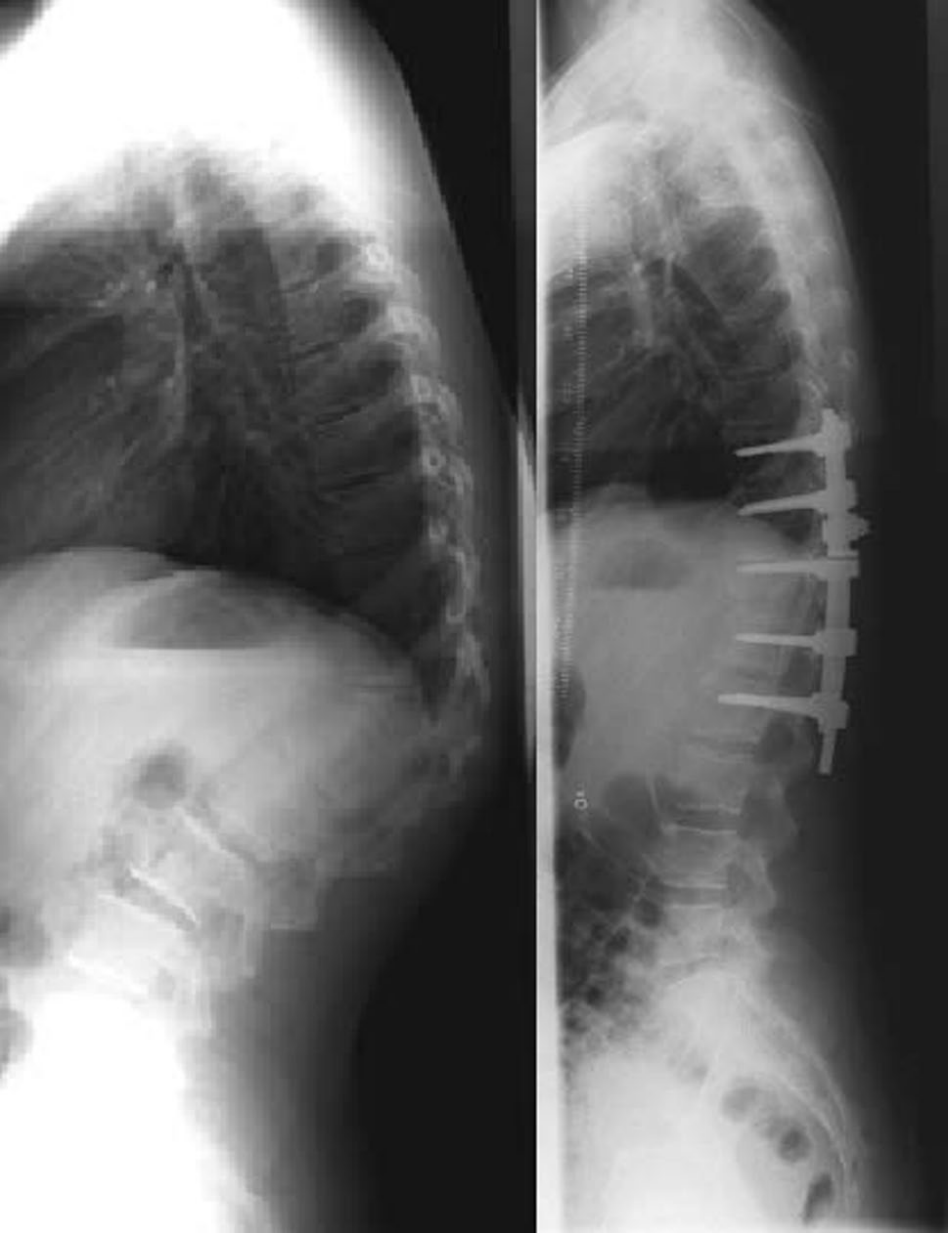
15-year-old boy with congenital kyphosis at the thoracolumbar junction. Standing lateral radiograph before and after Th12 PVCR and instrumentation from T9 to L2 at 2-year follow-up
